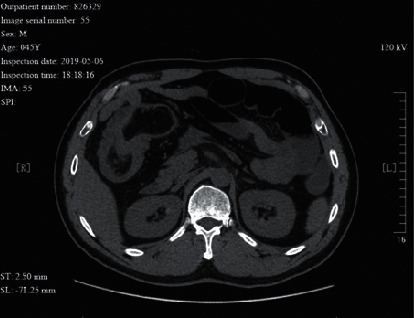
A 45 year-old male patient with intussusception caused by lipoma of the ileum. The CT image shows a cup mouth sign. No bowel wall ischemia was identified during surgery.
